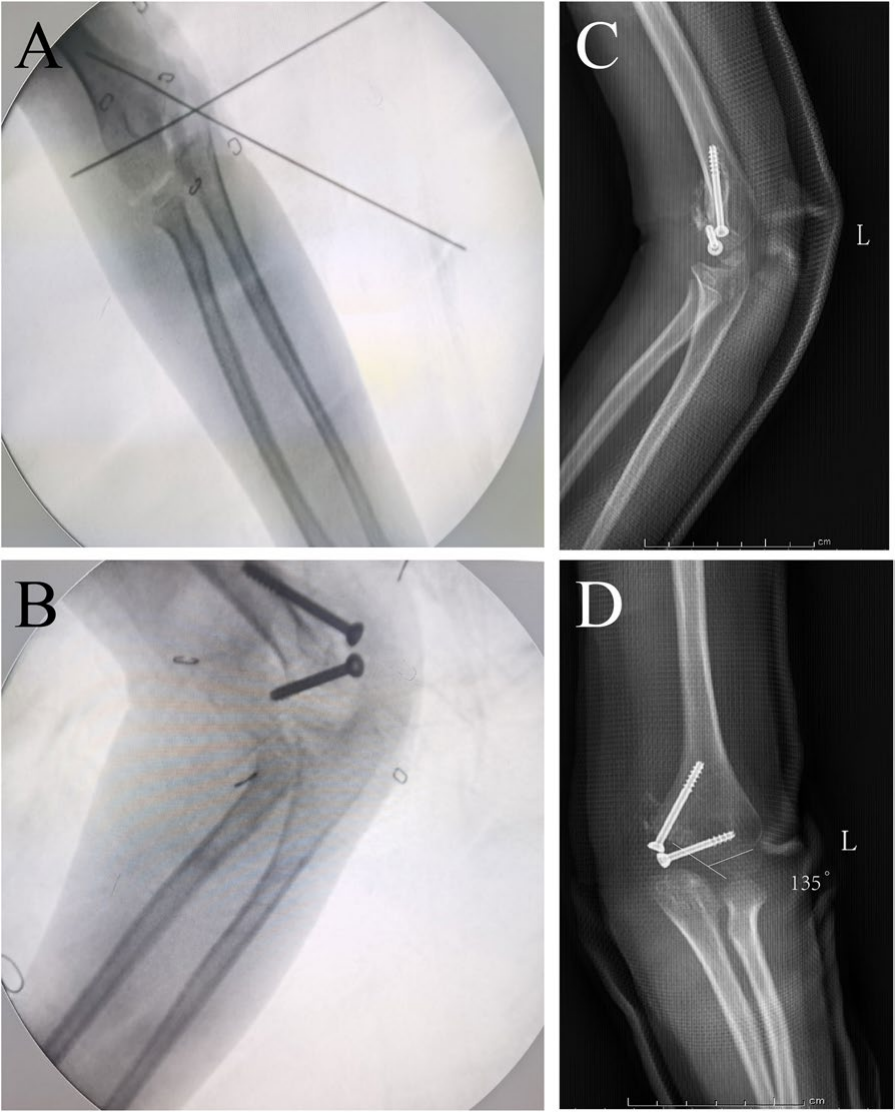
Intraoperative and postoperative X rays. A Using Kirschner wire for positioning. B Using metal screw fixation. C-D Postoperative X-ray appearance after cast immobilization
